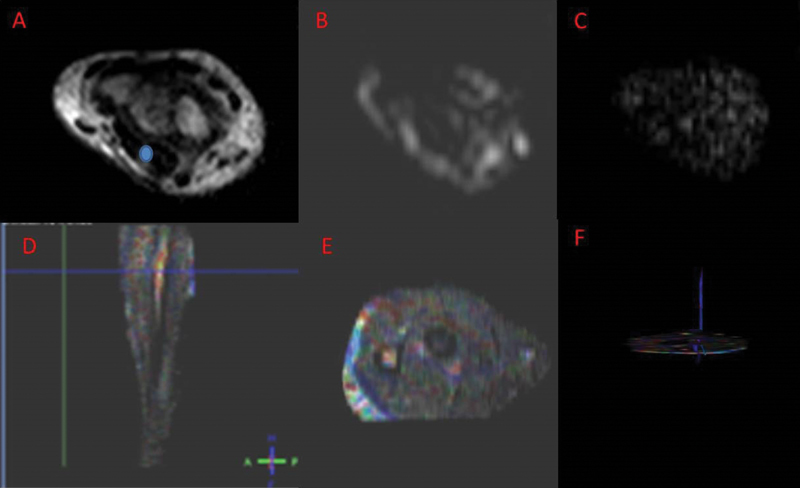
Right (peripheral nerve schwannoma) wrists of a 27-year-old female. T1-weighted magnetic resonance imaging (MRI;
